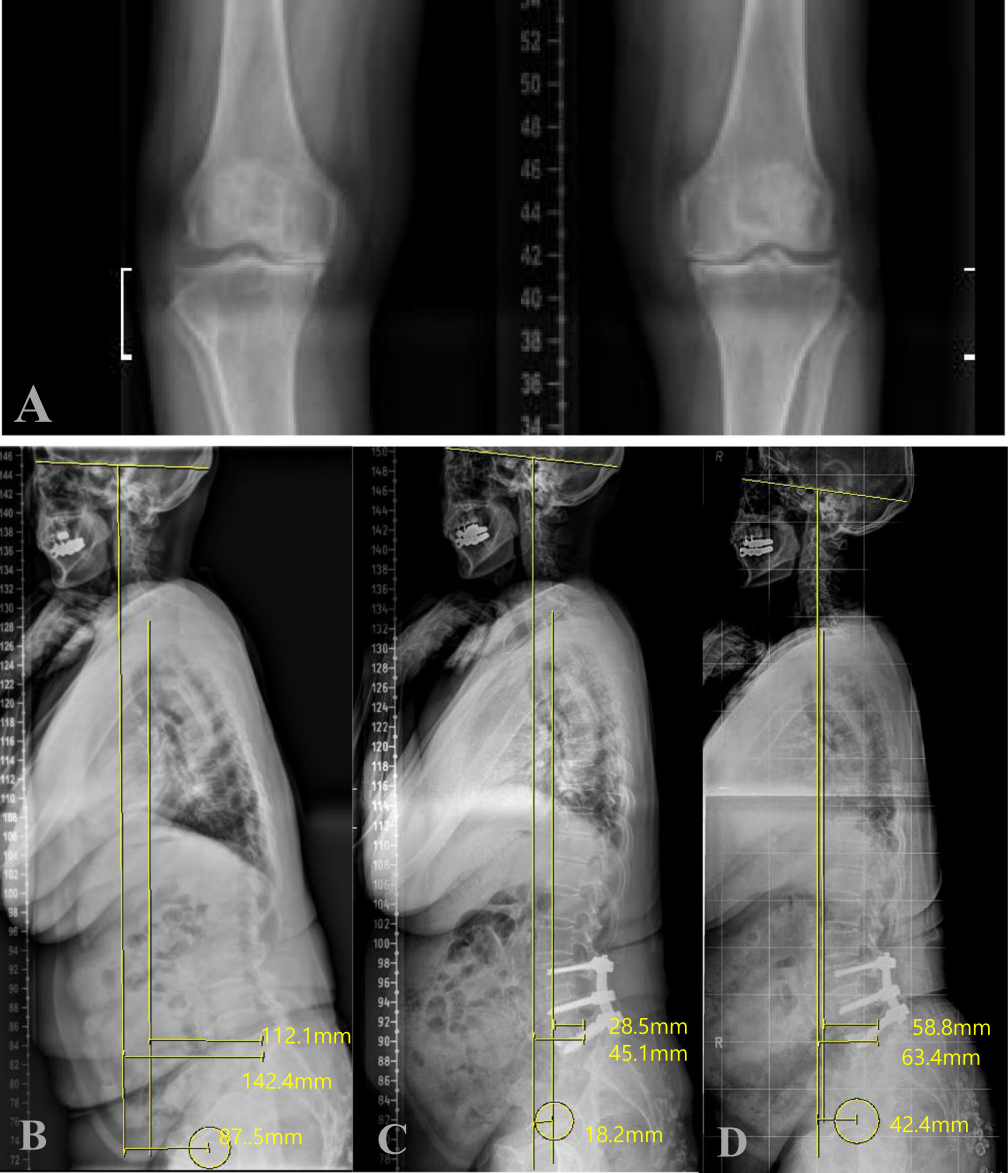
A case of 67-year old female patient, who underwent L3-L5 instrumented fusion. a Preoperative standing knee AP radiograph shows severe (KLG 4) knee OA. Whole spine stnading lateral radiographs taken at b the preoperative, c the postoperative 1 month, and d the postoperative 3-year follow-up show reaggravation of radiographic sagittal balance which was improved at postoperative 1 month
